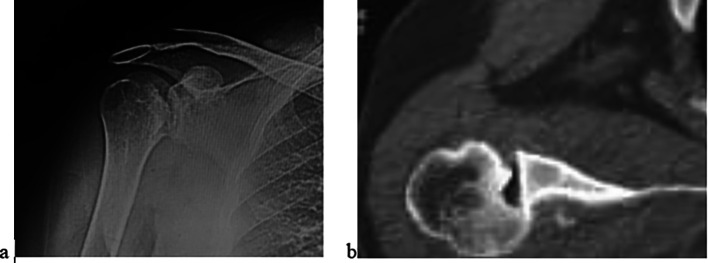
Preoperative imaging of right shoulder with locked posterior shoulder dislocation and reverse Hill-Sachs. (a) Anteroposterior X-ray radiograph and (b) Axial CT scan cut.
